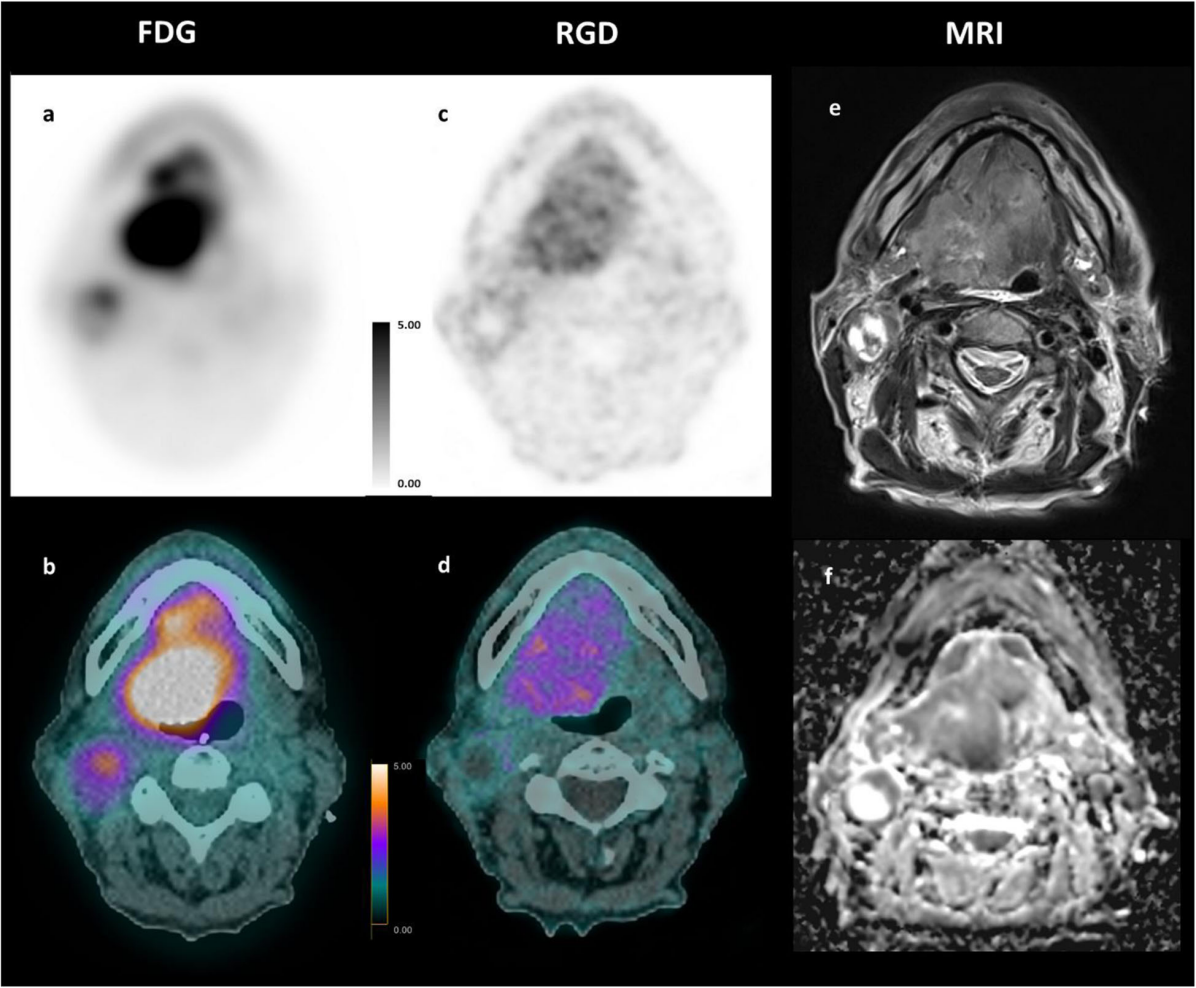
Comparative MRI, ^18^F-FDG, and ^68^Ga-NODAGA-RGD PET/CT of patient #8. Axial PET/CT fusion slices of a 69-year-old man with a moderate differentiated base tong SCC. The images show different tumor-to-background ratios in between the two radiotracers ^18^F-FDG PET/CT (a, b) vs. ^68^Ga-NODAGA-RGD PET/CT (c, d), and also a slightly different distribution of activity within the tumor bed when compared with the MR images e T2w and f ADC map of diffusion
